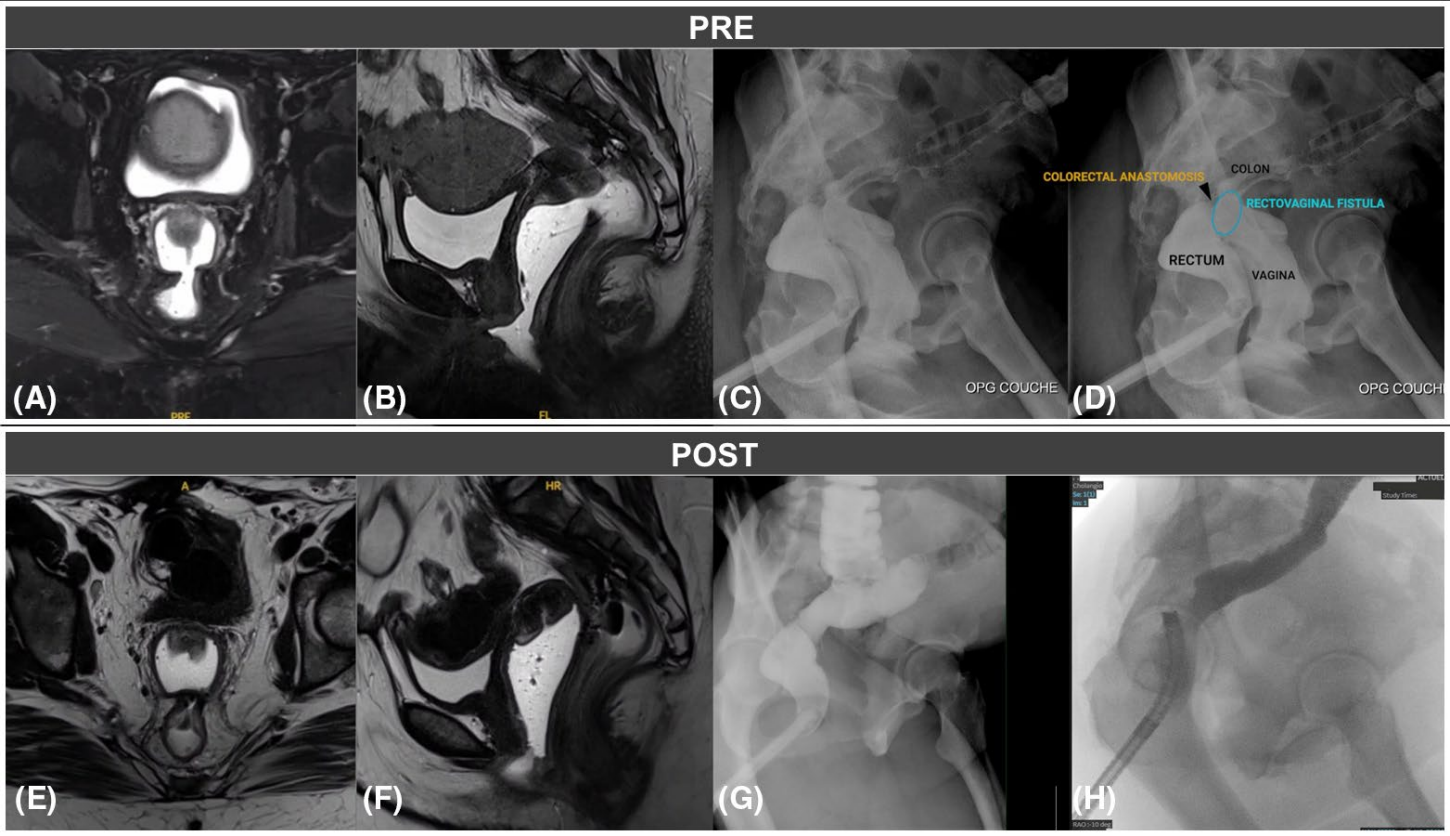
PRE* preoperative pelvic magnetic resonance imaging (MRI)—axial (A) and sagittal (B) views—and lower GI series with rectal opacification (C and D) clearly showing a wide communication between the anterior aspect of the colorectal anastomosis and the posterior vaginal fornix; *POST* postoperative pelvic MRI—axial (E) and sagittal (F) views—and lower GI series with rectal opacification (G and H) clearly showing a successful closure of the rectovaginal communication.
